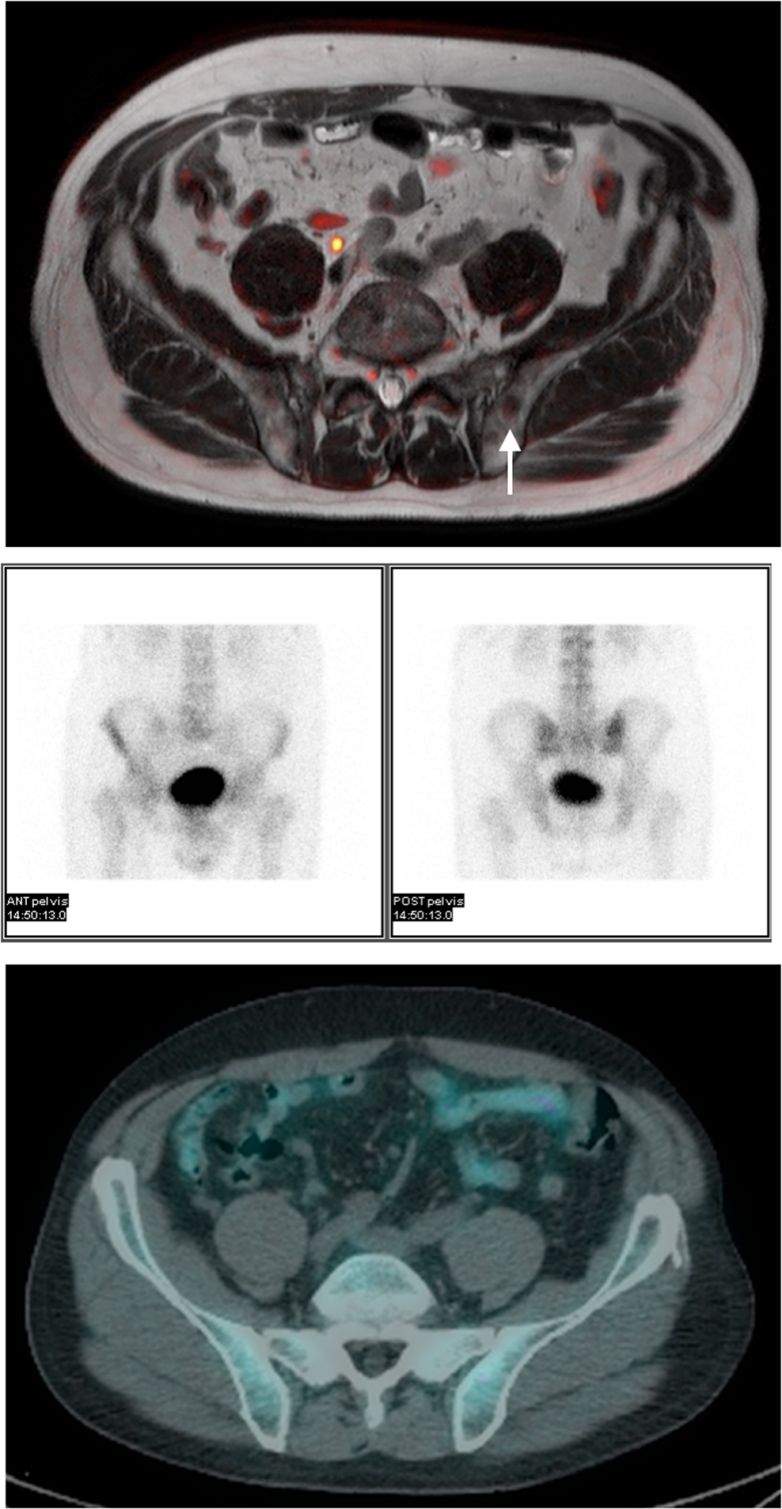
Example of a discordant skeletal site in a 73-year-old man with a serum prostate-specific antigen level of 19.4. Top: Axial T2-weighted turbo spin echo with fused *b* = 1000s/mm^2^ showing a metastasis in the left iliac body, which is occult on BS (middle) and ^18^F-choline PET/CT (bottom). The site was considered to represent a true positive whole-body MRI, false negative ^18^F-choline PET/CT, and false negative ^99m^Tc bone scintigram
